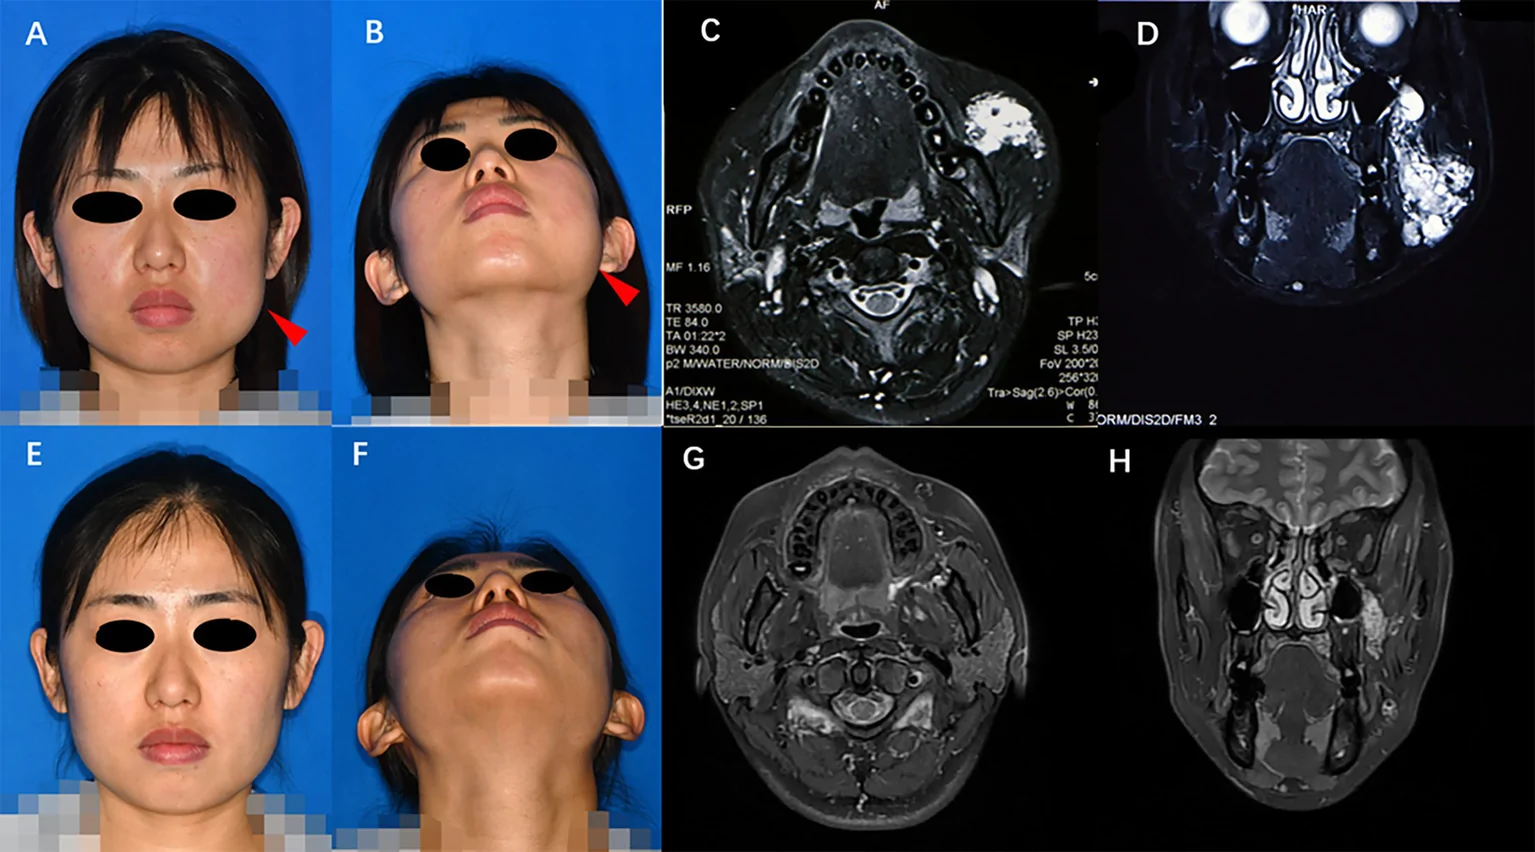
Representative type II lesion before and after treatment. (A,B) Pre-treatment clinical photographs show cheek fullness and facial asymmetry. (C,D) Pre-treatment MR images show extension of the lesion into adjacent deep facial spaces. (E,F) Post-treatment clinical photographs show improved facial symmetry. (G,H) Post-treatment MR images show decreased lesion extent.
